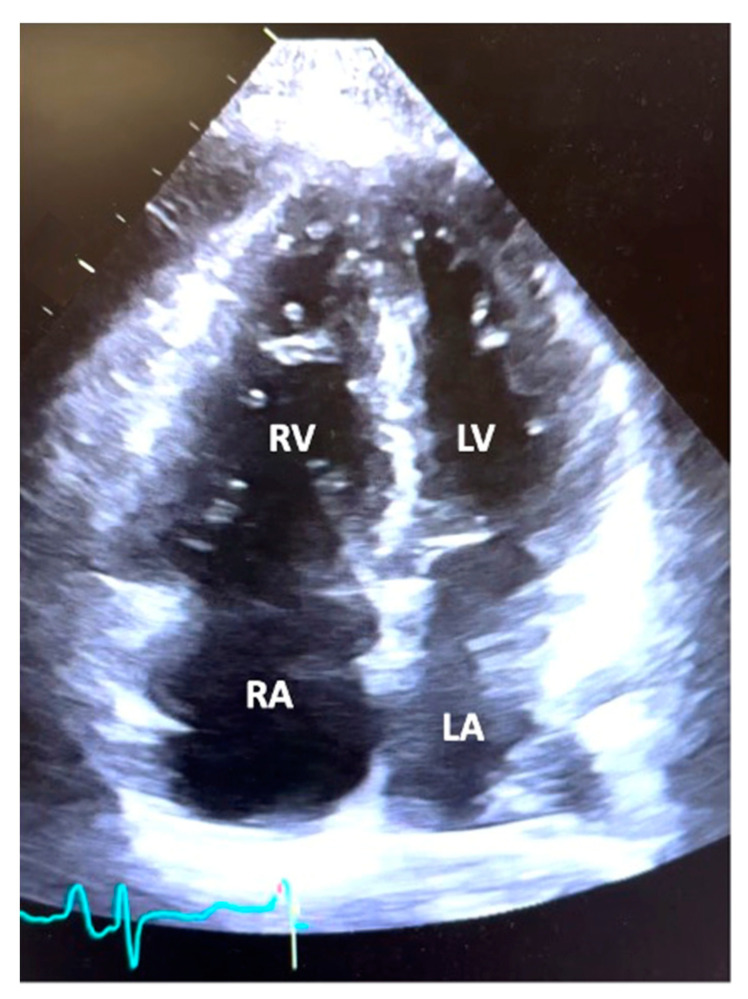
Echocardiogram. A representative image of an apical four-chamber view from a patient in the study demonstrating moderate right ventricular (RV) dilation, right atrial (RA) dilation, and normal left ventricular (LV) and left atrial (LA) size.
